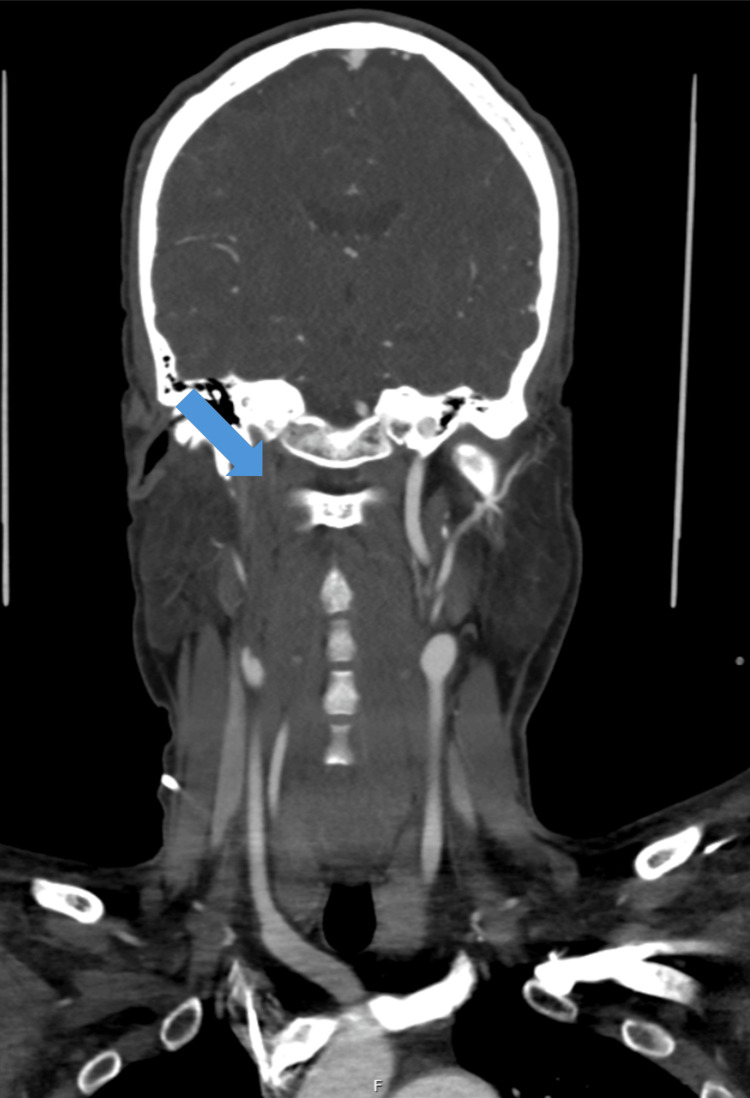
Brain CTA in the emergency room showing the total obliteration (blue arrow) of the right internal carotid artery CTA: computed tomography angiography
